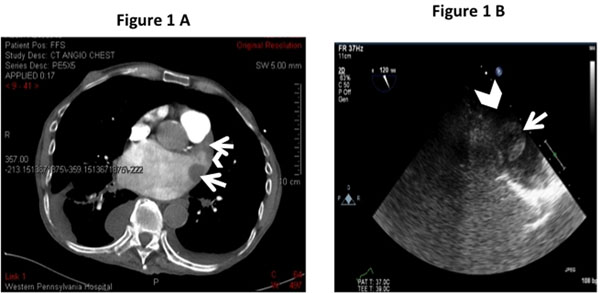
A. CT angiogram of the chest showing left atrial/left atrial appendage (arrow head) and masses (arrows) Figure 1 B. Transesophageal echocardiogram showing left atrial appendage (arrow head) and thrombus (arrow)
